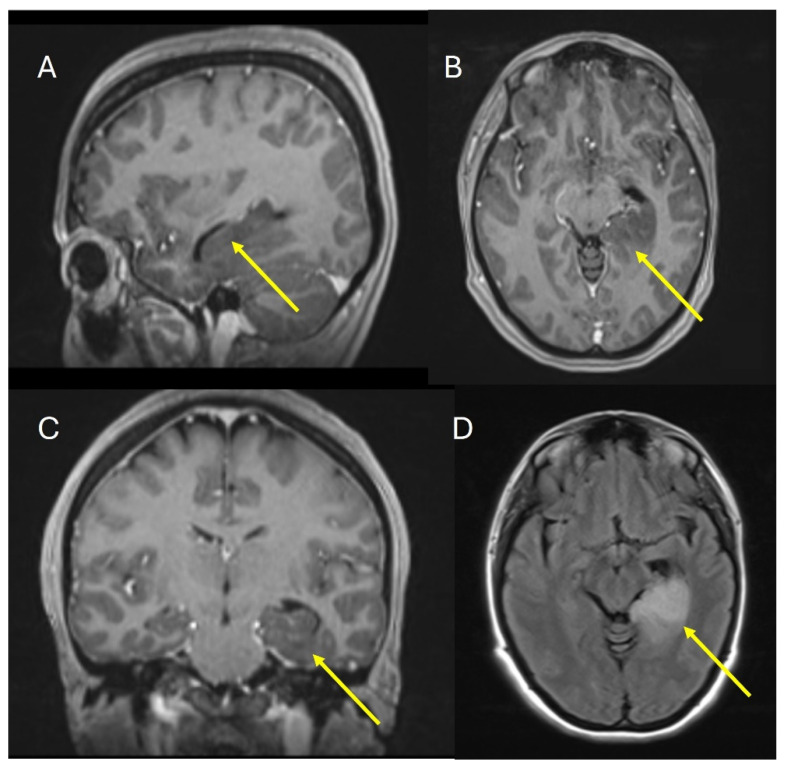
MRI of the brain. (A) Saggital image T1WI, (B) Axial image T1WI, (C) Coronal image T1WI, (D) FLAIR axial image. The yellow arrow shows the hippocampal formation and parahippocampal gyrus.
